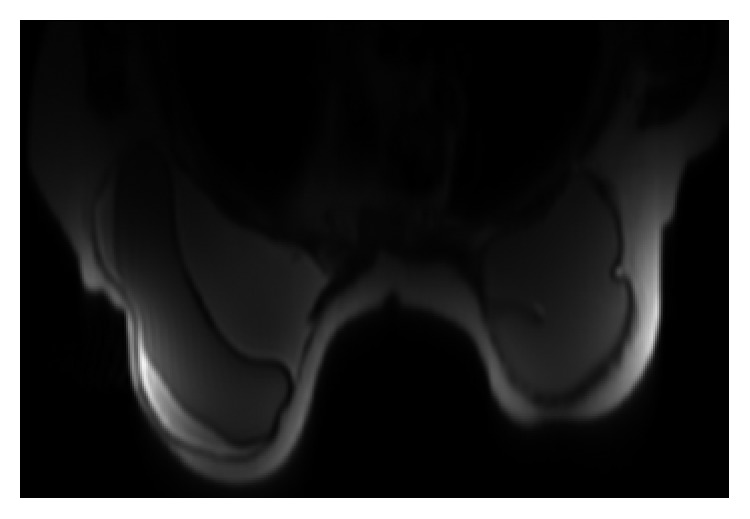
MRI showing fluid accumulation around the left breast subpectoral silicone implant.
